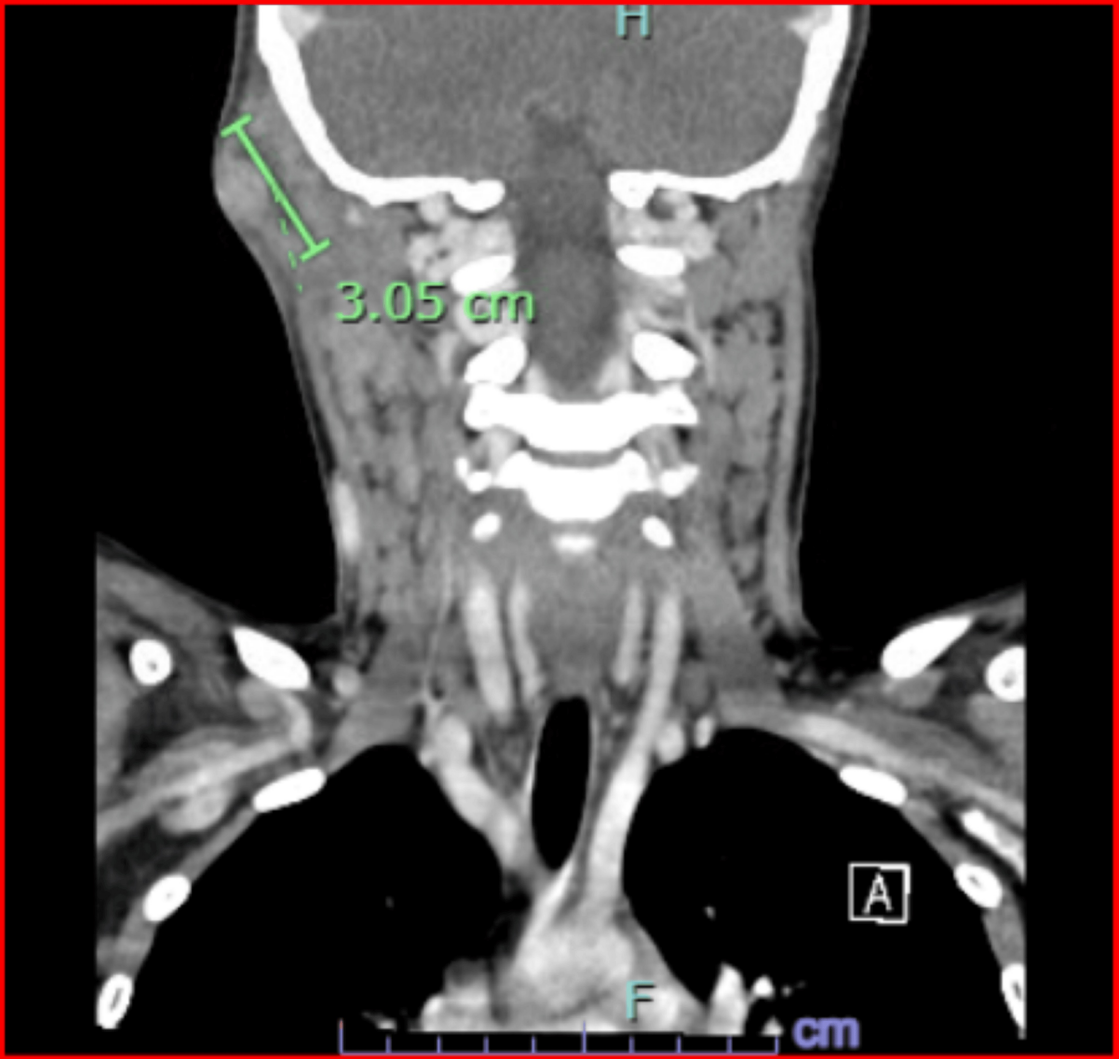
CT of the soft tissue neck with contrast. CT shows a mixed-attenuation soft tissue abnormality in the right posterior auricular area of the upper neck adjacent to the posterior margin of the mastoid bone that indicates suppurative or abscessed lymph node within the central low-density component measuring 3.05 cm in diameter. The mastoid air cells and middle ear cavities are clear. Additional enlarged lymph nodes are identified in the sub-adjacent right posterior triangle of the suprahyoid neck and mild lymphadenopathy in the left posterior triangle of the suprahyoid neck (not highlighted in the image).
